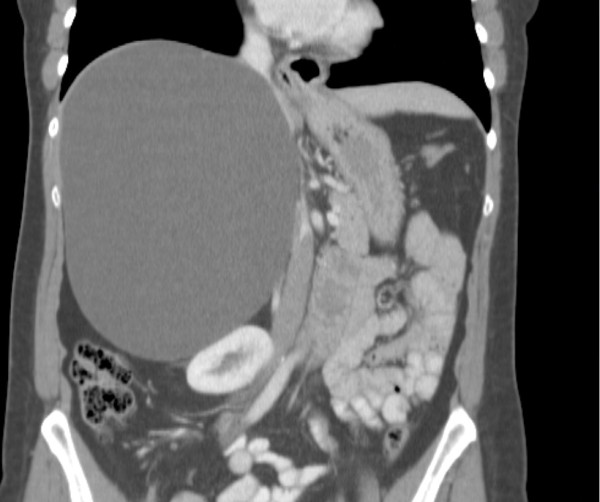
CT scan showing the 20 x 14 cm simple liver cyst.
